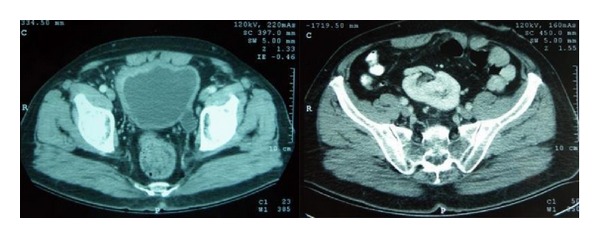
Preoperative CT of case  1: bladder carcinoma preoperative and left pelvic kidney.
